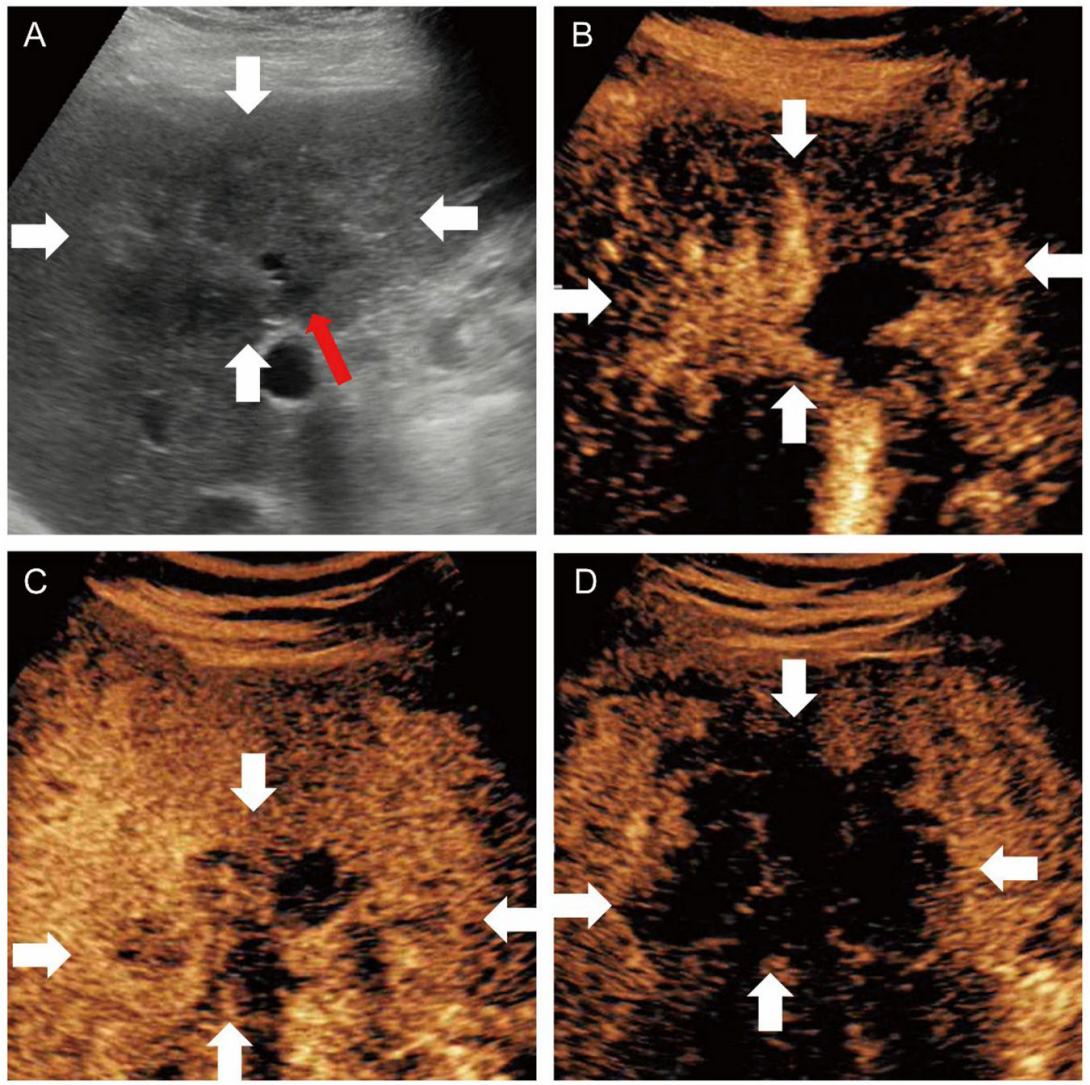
A 76-year-old female with a 56-mm biliary type MF-ICC. B-mode ultrasound showed hypo-echogenicity lesion and dilated bile duct (A). Non-circular enhancement was observed in the arterial phase (B). Hypo-enhancement was observed in the portal venous phase (C) and late phase (D). White arrow indicated the margin of the lesion; red arrow indicated the dilated bile duct
